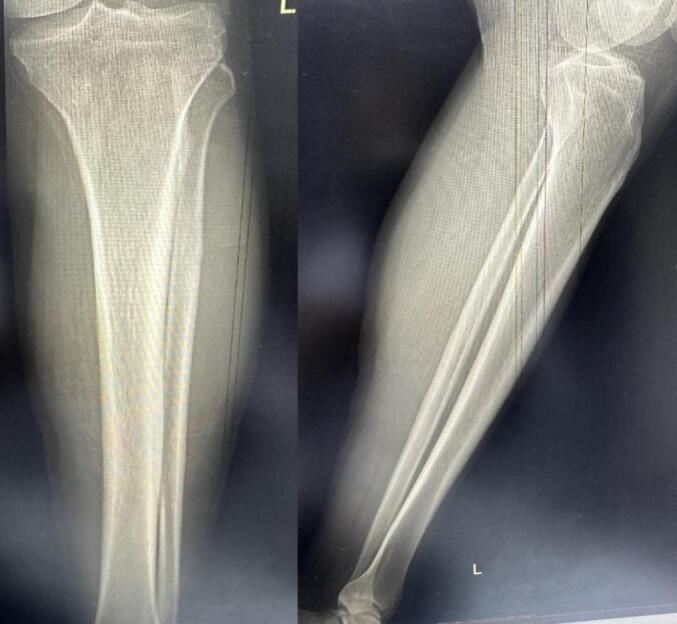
Standard radiographs of the leg revealed no abnormalities.
